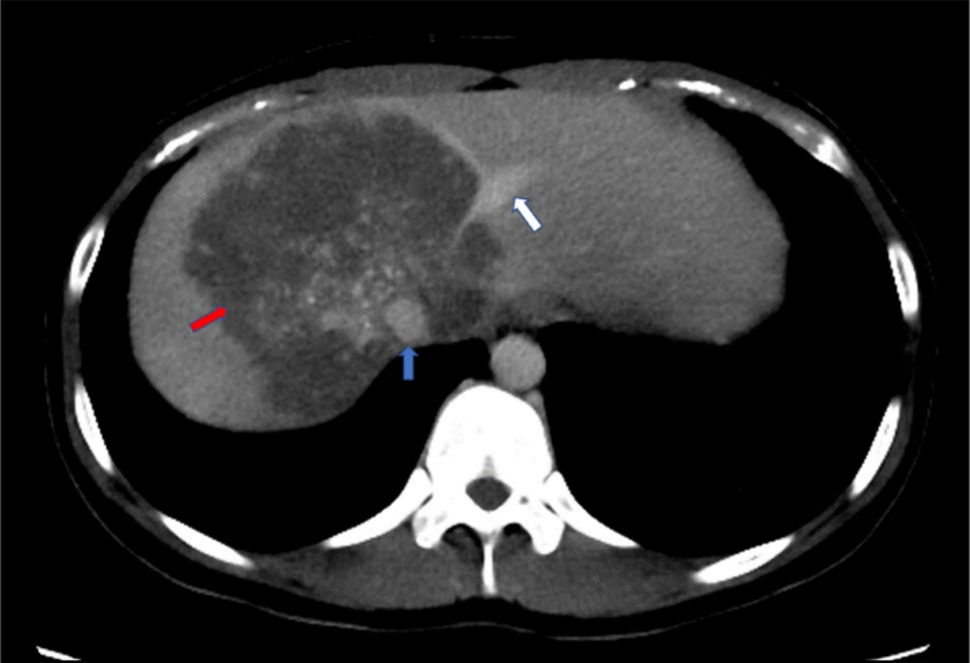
A 23-year-old female patient with HAE. Axial CT imaging in the portal venous phase showed HAE lesion (red arrow) had invaded the secondary porta hepatis, with absence of the RHV and MHV, the LHV (white arrow) was with lumen stenosis and irregular wall, and the lesion-vessel contact angle of IVC (blue arrow) was grade 4. Pathological examination confirmed the invasion of RHV, MHV, LHV and IVC. RHV, right hepatic vein; MHV, middle hepatic vein; LHV, left hepatic vein; IVC, inferior vena cava
